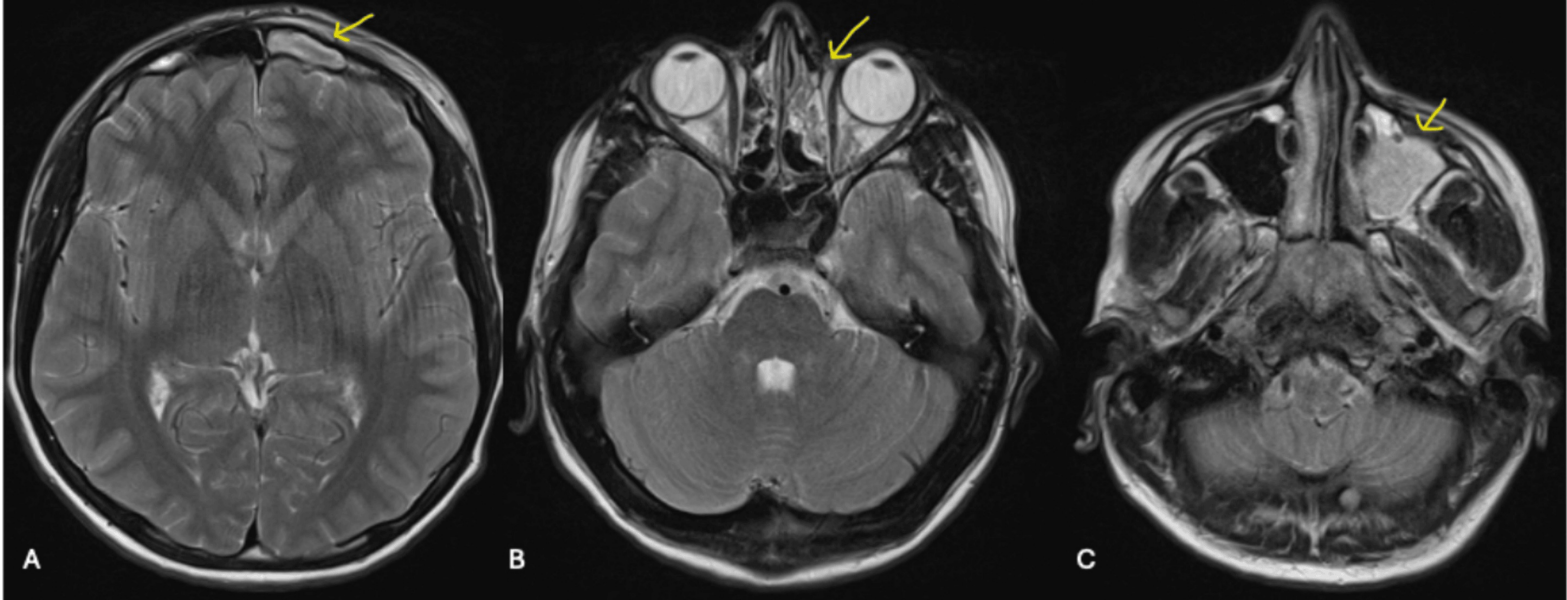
T2-weighted non-contrast MRI head and sinus in the axial view. A: Left frontal sinus occupied (arrow). B: Left ethmoidal cells occupied (arrow). C: Left maxillary sinus occupied (arrow).
